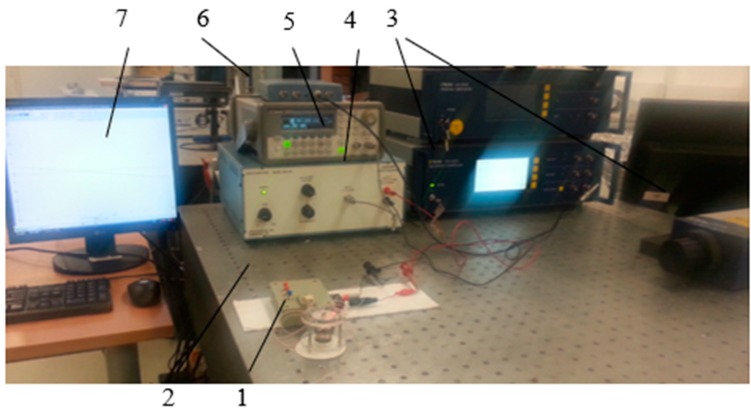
Experimental setup for measurement of resolution of the angular rotation: 1—USM with spherical rotor; 2—controller; 3—Polytec Laser Doppler Vibrometer system OFV512/5000; 4—linear amplifier EPA-104; 5—signal generator Agilent 33220A; 6—oscilloscope PicoScope 3424; 7—PC with a PicoScope and Polytec software.
